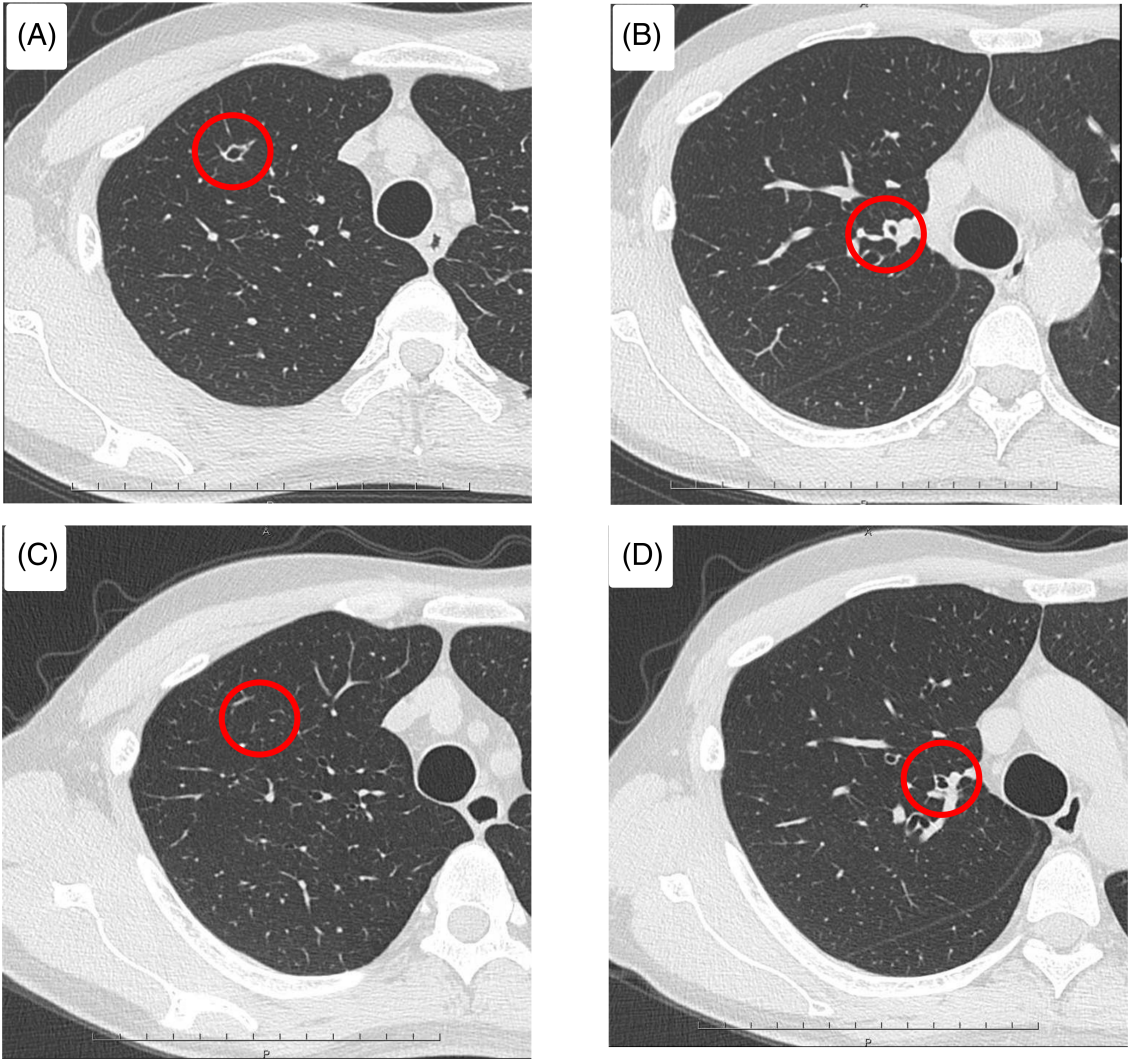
(A, B) High‐resolution computed tomography scan revealing focal bronchiectasis and bronchial wall thickening in the right upper lobe. (C, D) Bronchial wall thickening improved following treatment with fluconazole.
